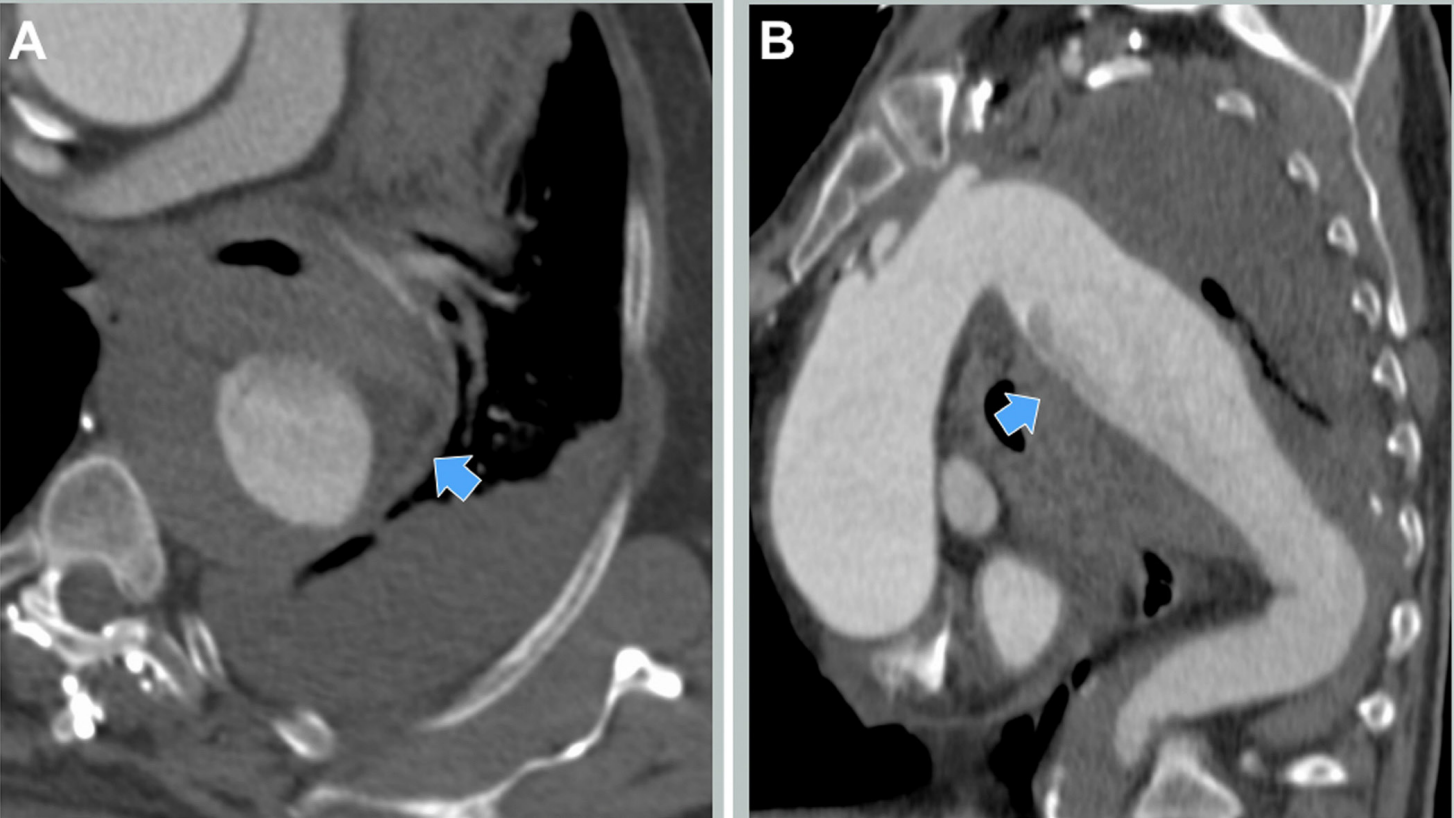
Preoperative CT-scan. (A) Axial view with periaortic hematoma (arrow); (B) Sagittal view with the possible point of descending thoracic aortic rupture indicated (arrow).
